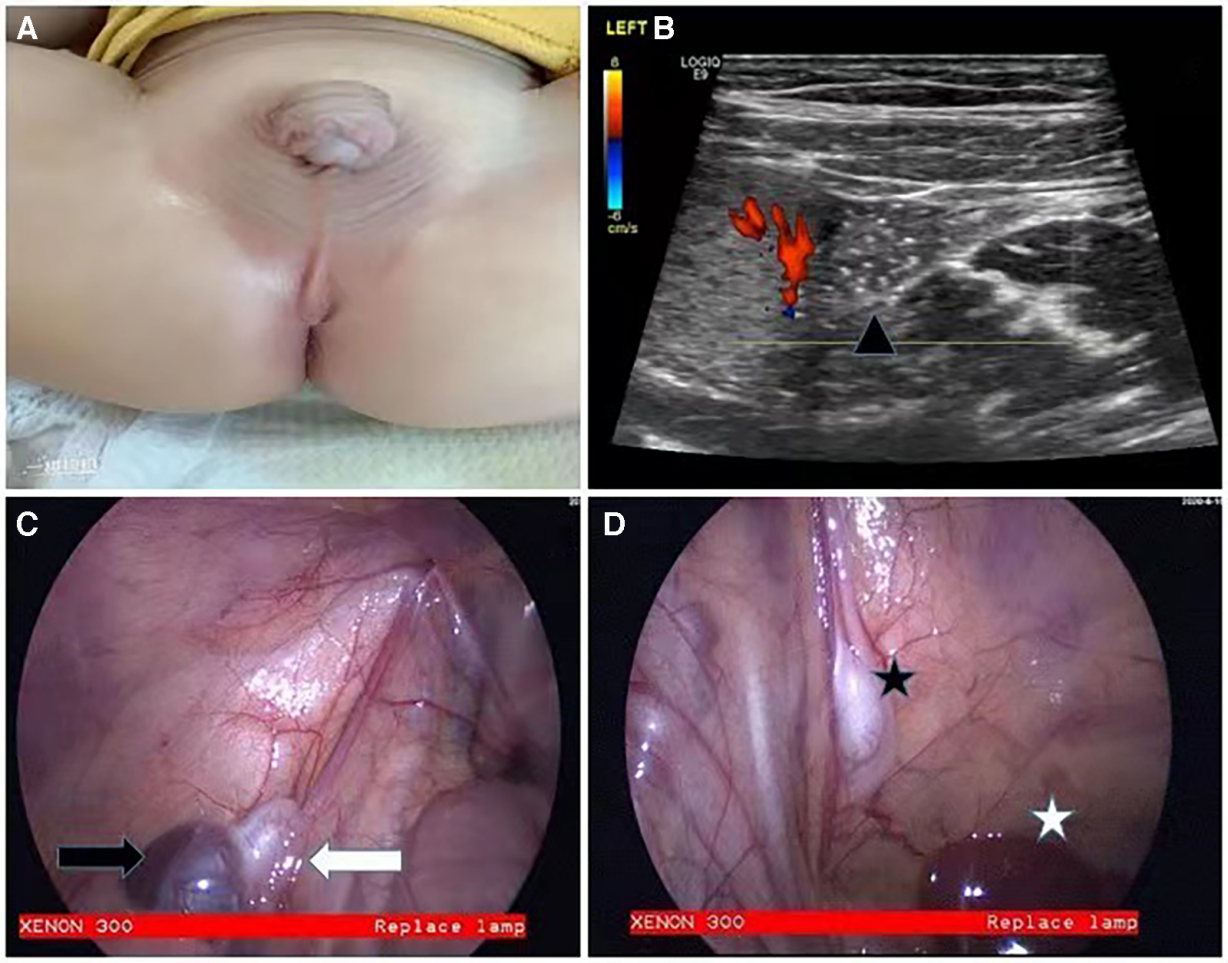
Appearance of case 1 and intraoperative findings. Physical examination showed bilateral impalpable testes and coronal hypospadias (A). Preoperative ultrasound suggested that the left testis was adjacent to the lower pole of the spleen (B). The left testis was fused to the spleen (C), and the right testis was located in a flat umbilical position at the lower edge of the liver (D) (Black triangle: left testis; black arrow: spleen; white arrow: left testis; black star: right testis; white star: liver.)
